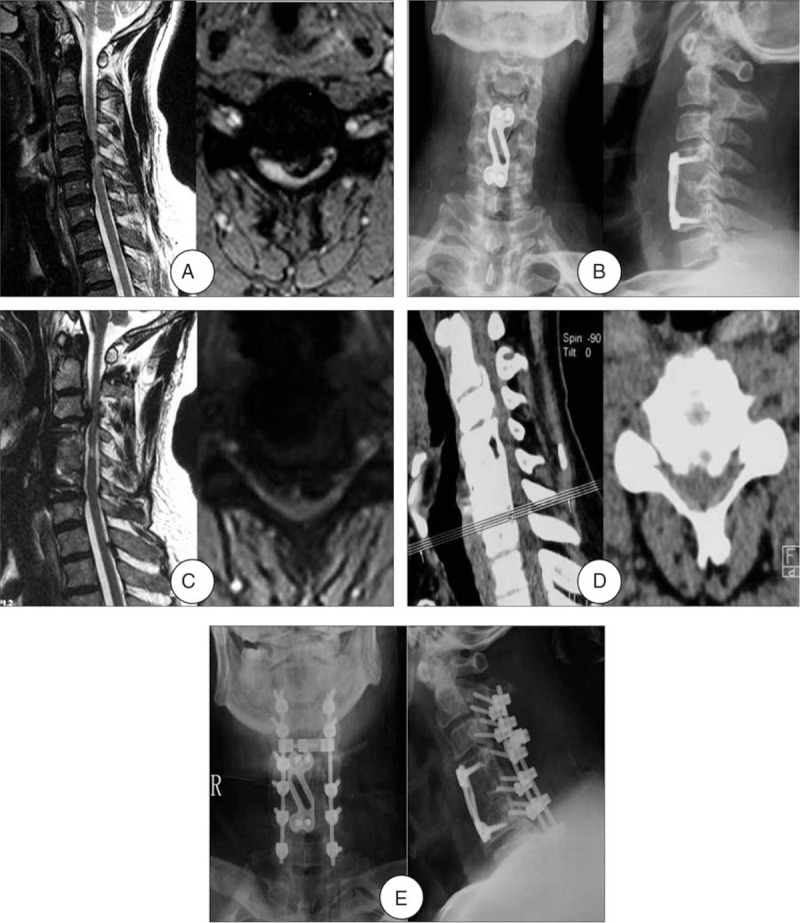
A 66-year-old man developed multi-level ASD 11 years after initial surgery. (A) Preoperative MRI of this patient shown severe compressions of the spinal cord at C4-5 level as well as high signal intensity in the spinal cord. (B) Radiograph after initial surgery shown ACCF at C4. (C) and (D) MRI and CT at 11-year follow-up shown development of ASD and spinal cord compression at C2-3, C3-4, and C6-7. (E) Radiograph after the reoperation shown laminectomy with internal fixation. ACCF = anterior cervical corpectomy and fusion; ASD = adjacent segment disease, CT = computed tomography, MRI = magnetic resonance imaging.
